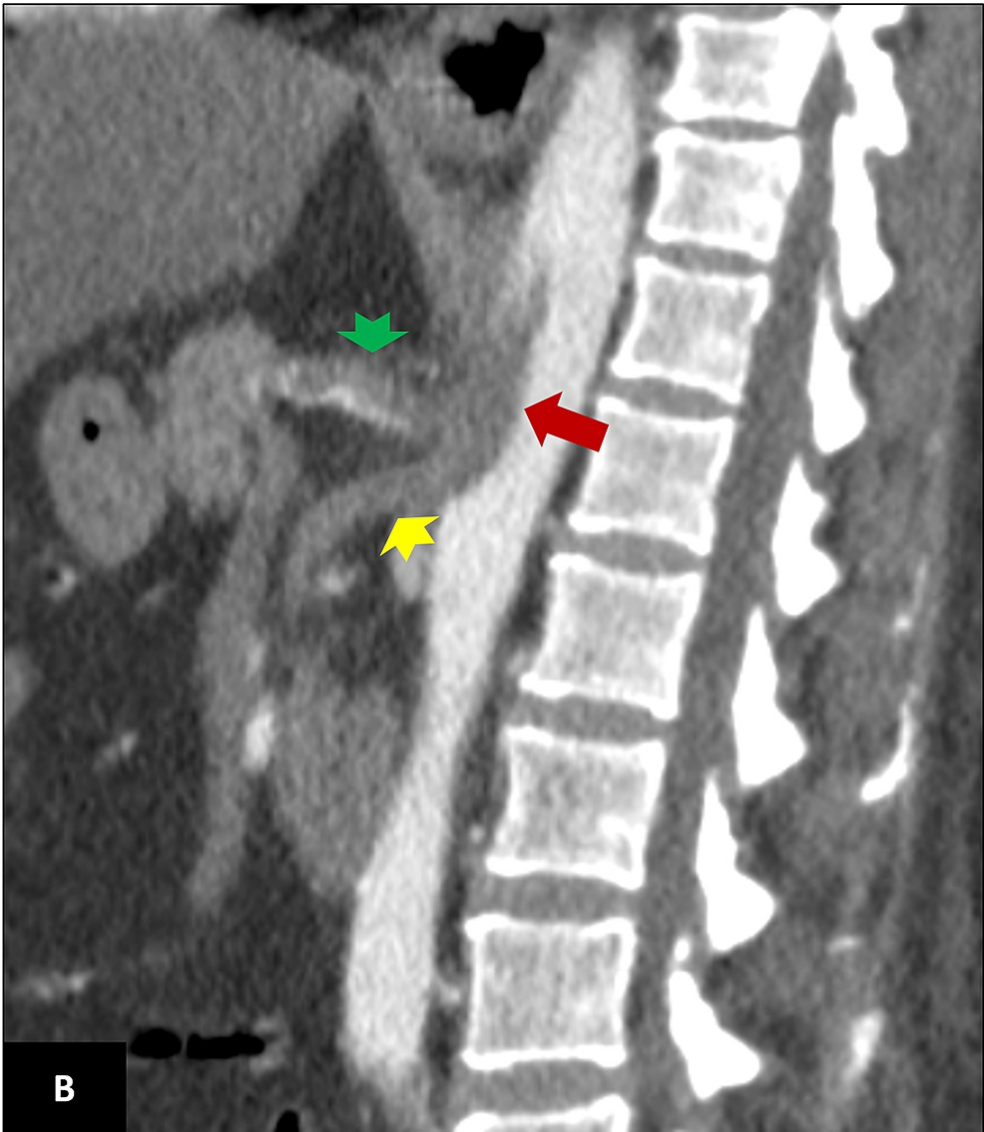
Sagittal contrast-enhanced CT scan of the same patient showing a large thrombus (red arrow) extending from the abdominal aorta and almost completely occluding the celiac trunk (green arrow head) and the superior mesenteric artery (yellow arrow head).
